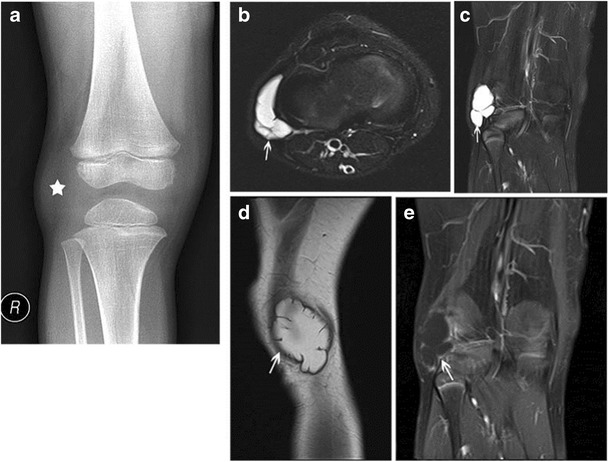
Ganglion cyst at the lateral margin of the right knee in a 6-year-old boy. An AP radiograph of the right knee a reveals slight soft-tissue fullness along the lateral aspect of the knee (*marked by star*). This corresponded to the site of fluctuant mass noted on palpation. No associated adjacent bony defects/erosive changes are observed. Axial b and coronal c fat-suppressed T2-weighted MR images show a lobulated, deformable, cystic mass adjacent to and wrapping around the lateral distal femoral metaphysis (*arrows*). A sagittal proton density image d reveals high signal within the fluid (due to high mucopolysaccharide content); thin rim enhancement was noted with contrast (*arrow*) ePeripheral rim-like gadolinium contrast enhancement can be seen, or there can be complete lack of enhancement. Usually, no solid/enhancing components are seen; although, occasionally, internal T2 hypointense debris or even osseous loose bodies can be seen [19]. Erosion/invasion of adjacent bone may be present.Haemorrhage or infection may occur within these lesions, resulting in wall-thickening and a heterogeneous internal signal on MR. Adjacent oedema and fluid tracking can be seen with cyst rupture [19].b.Post-traumaticChronic avulsive injuries/cortical desmoid:Cortical desmoids are usually a consequence of an avulsive injury or stress reaction at the insertion site of the medial head of the gastrocnemius muscle or adductor magnus.On plain films, these appear as small radiololucent saucer-shaped lesions or areas of cortical irregularity with an associated sclerotic base, typically at the posteromedial aspect of the distal femoral condyle (Fig. 11).
